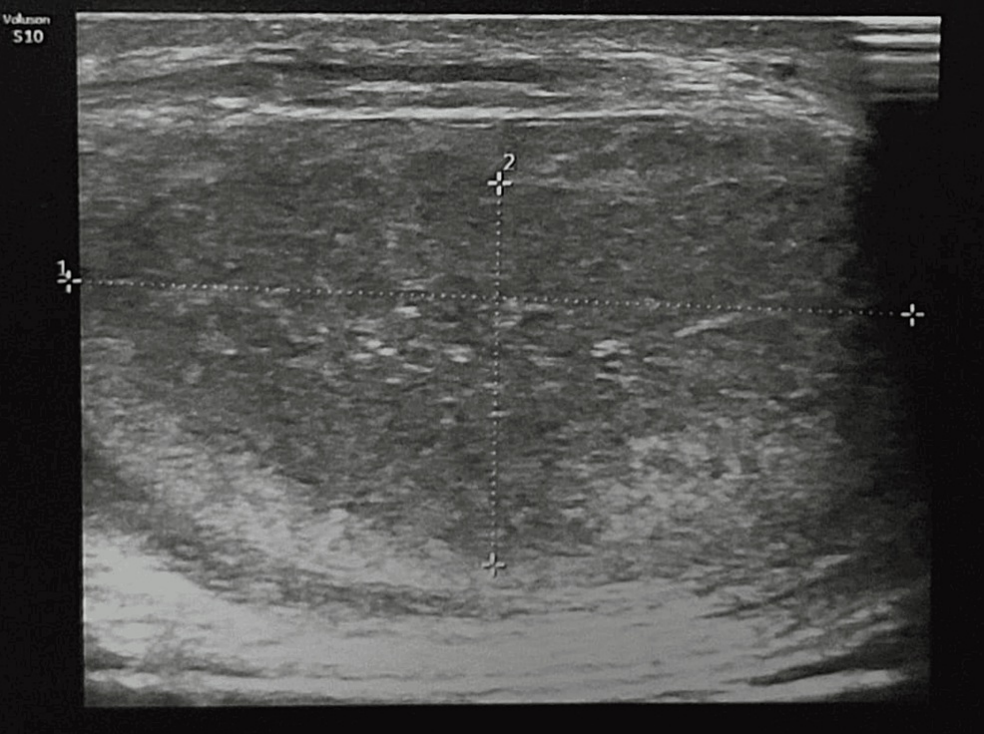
Patient's scrotal ultrasound A left scrotal ultrasound suggested a suspicious-looking mass.
